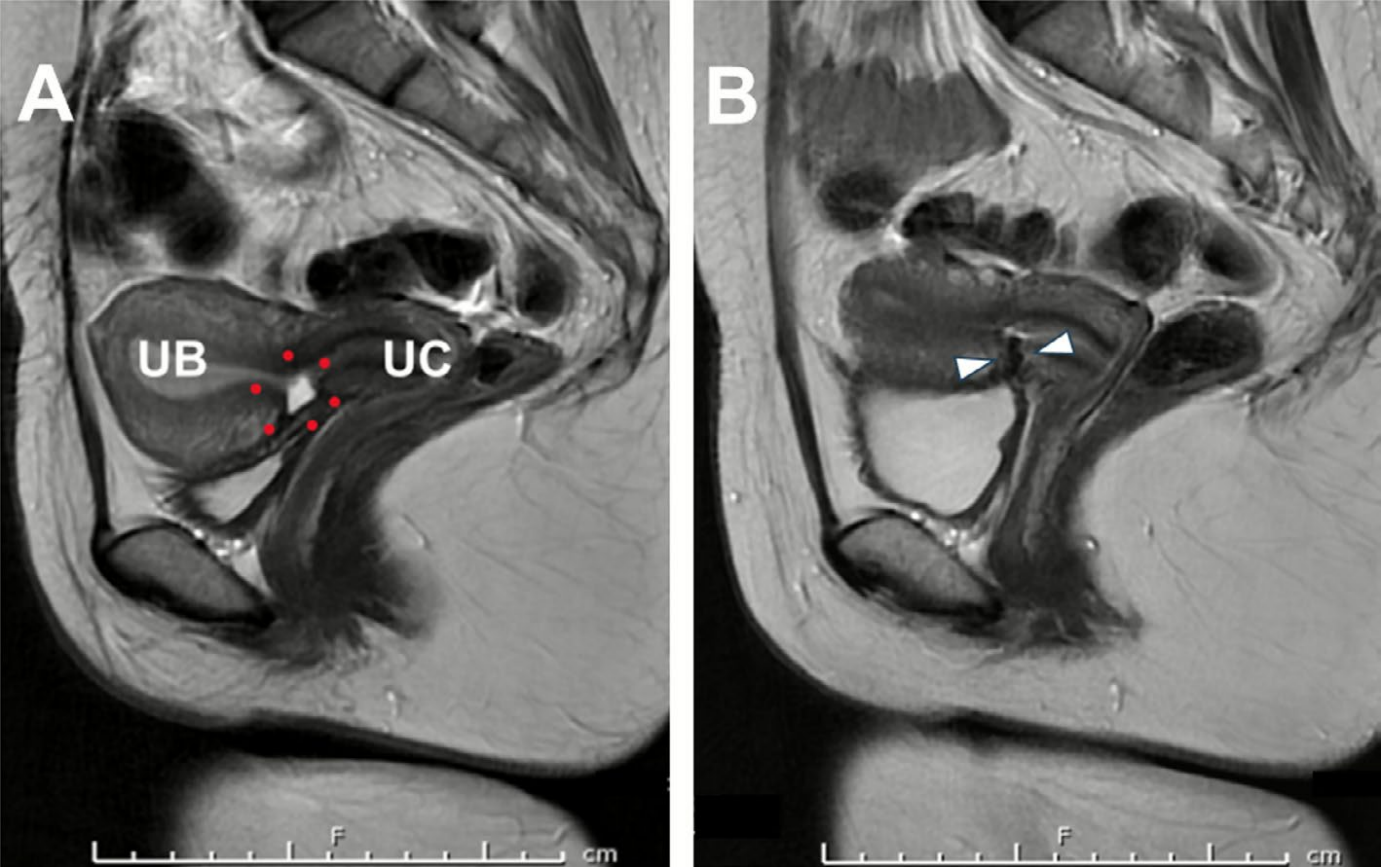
Preoperative and postoperative magnetic resonance imaging (MRI) findings. (A) Sagittal T2‐weighted image obtained from preoperative non‐contrast MRI. The anatomical landmarks are labeled as follows: uterine body (UB), uterine cervix (UC), and the cesarean scar niche, the latter being delineated by a red dotted outline. Consistent with the sonographic findings, the MRI delineated a pronounced niche formation accompanied by substantial thinning of the residual myometrium. (B) Sagittal T2‐weighted image obtained from postoperative non‐contrast MRI. The region corresponding to the cesarean scar niche observed in Image A is indicated by a white arrow; however, the niche has completely resolved, and the integrity of the myometrium is restored.
